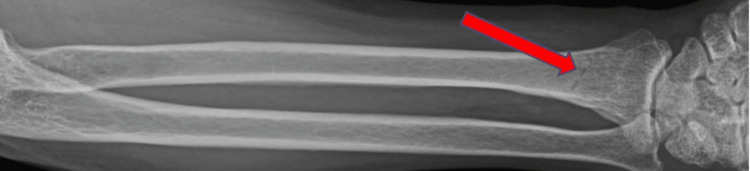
X-ray of a radial fracture from a fall during alcohol intoxication.
